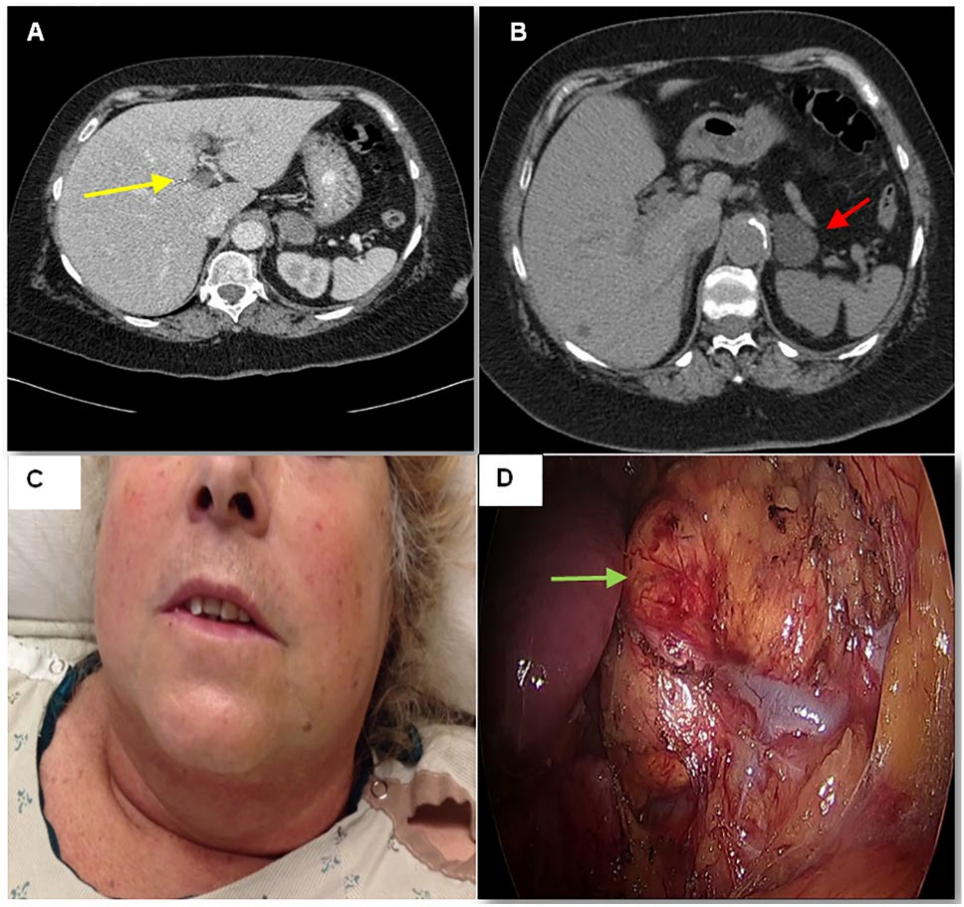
(A) Abdominal CT demonstrating left intrahepatic portal vein thrombosis (yellow arrow). (B) Noncontrast CT image of the left adrenal nodule revealing low attenuation and homogeneous appearance (red arrow). (C) Photograph of the patient’s head and neck demonstrating moon facies, plethora, and mild hirsutism. (D) In situ view of the left adrenal nodule prior to surgical resection (green arrow).
